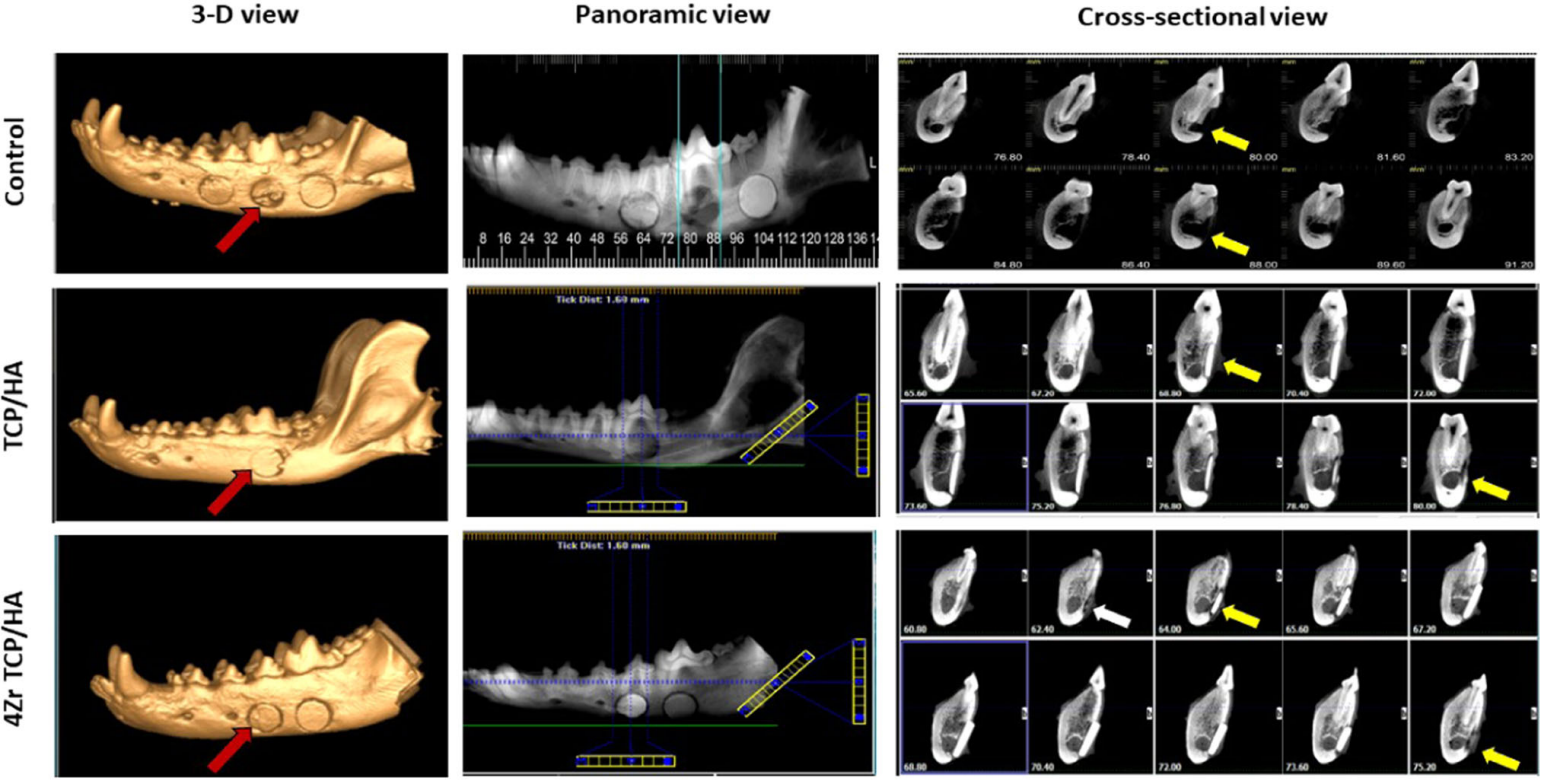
Three dimensional, panoramic and cross-sectional view cone-beam computed tomography of the induced mandibular defects at 12 weeks examination. Control defects (red arrow) demonstrated radiolucent circular defect with limited peripheral radiopacity without buccal rim corticalization (yellow arrow) around inferior alveolar canal (IAC). Defects grafted with tricalcium phosphate hydroxyapatite scaffolds (TCP/HA) (red arrow) demonstrated reduction in the size of the defect that was bounded by new bone formation with corticalization of the buccal rim (yellow arrow) around IAC. Defects grafted with zirconia-doped tricalcium phosphate hydroxyapatite scaffolds (4Zr TCP/HA) (red arrow) demonstrated reduction intimate contact with new bone formation with buccal rim corticalization around IAC (yellow arrow) with subperiosteal new bone formation (white arrow)
